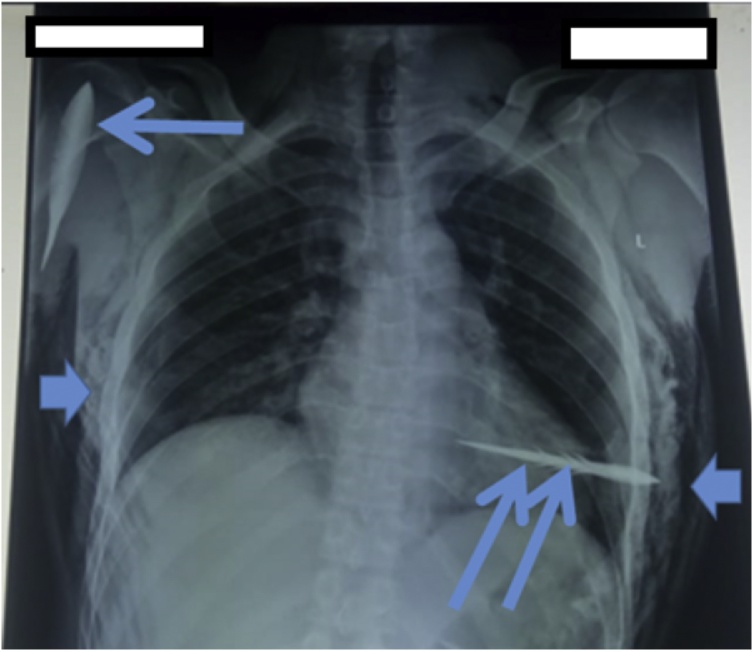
Chest radiograph of the patient; Single long arrow - arrow head superimposed on humeral head; Two long arrows - Arrow superimposed on cardiac silhouette and lung field; Short arrows - emphysema over right and lateral chest walls.
